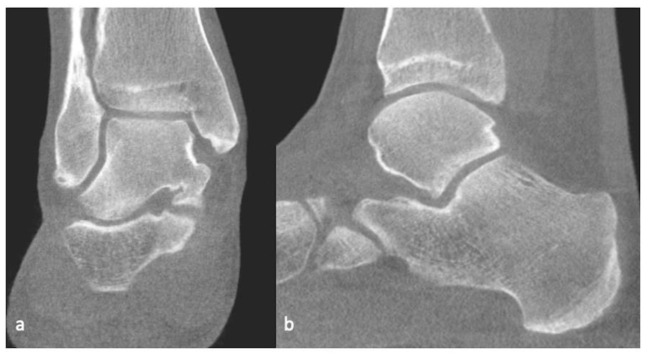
Weight-bearing CT, coronal (a) and sagittal (b) planes of the ankle.
